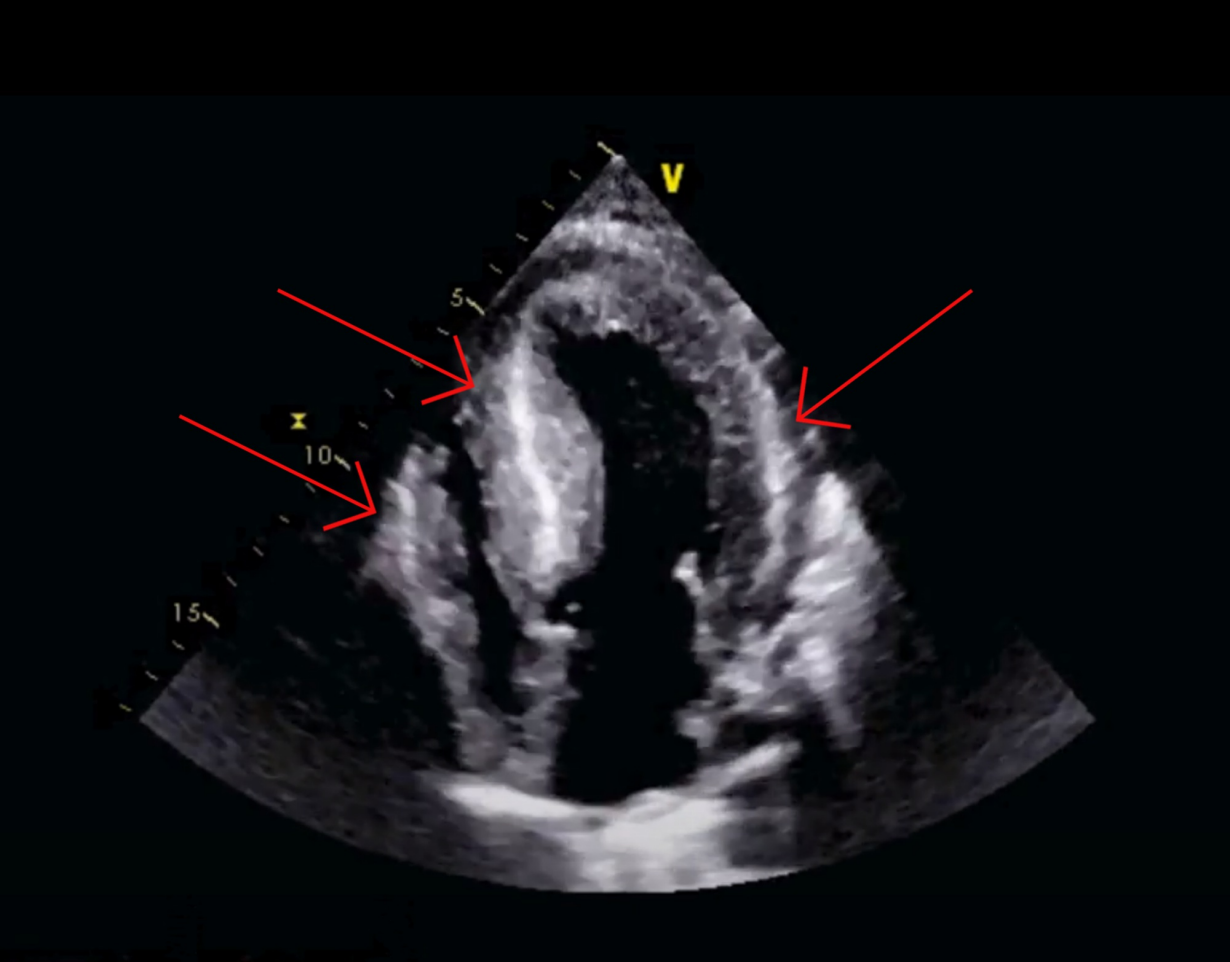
Echocardiogram showing thickened ventricular walls (arrows)
